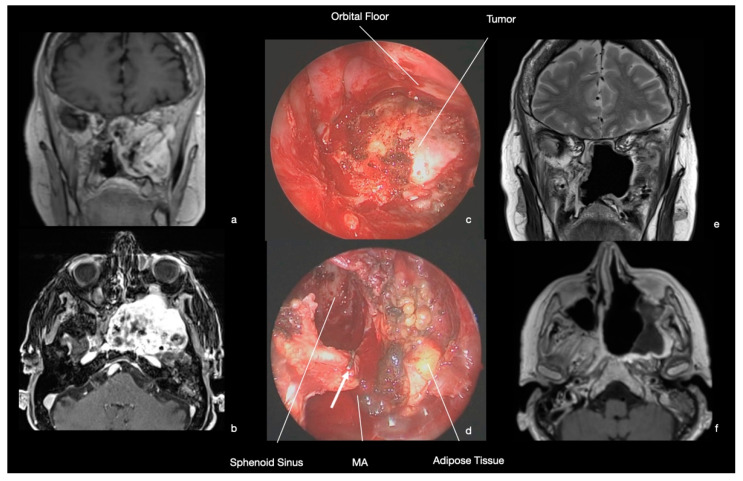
MRI and intraoperative endoscopic images (0° scope). (a,b) MRI. T1 w-sequence with contrast agent. A large mass extending in the left PPF and ITF was demonstrated. The floor of the orbit was pushed superiorly, with no suspect of periorbit infiltration. (c) Intra-operative image. After medial maxillectomy and resection of the posterior wall of maxillary sinus, the soft tumor was progressively resected. It was possible to cleave the lesion from the orbital floor. (d) Intra-operative image. At the end of the surgery, the tumor was completely resected with preservation of PPF anatomical structures. The maxillary artery (MA) has been identified and clipped (white arrow) during the tumor removal. (e,f) MRI. Coronal and axial view. T2 w-sequence and T1 w-sequence with contrast agent. Radical tumor resection was confirmed with no surgical complications.
